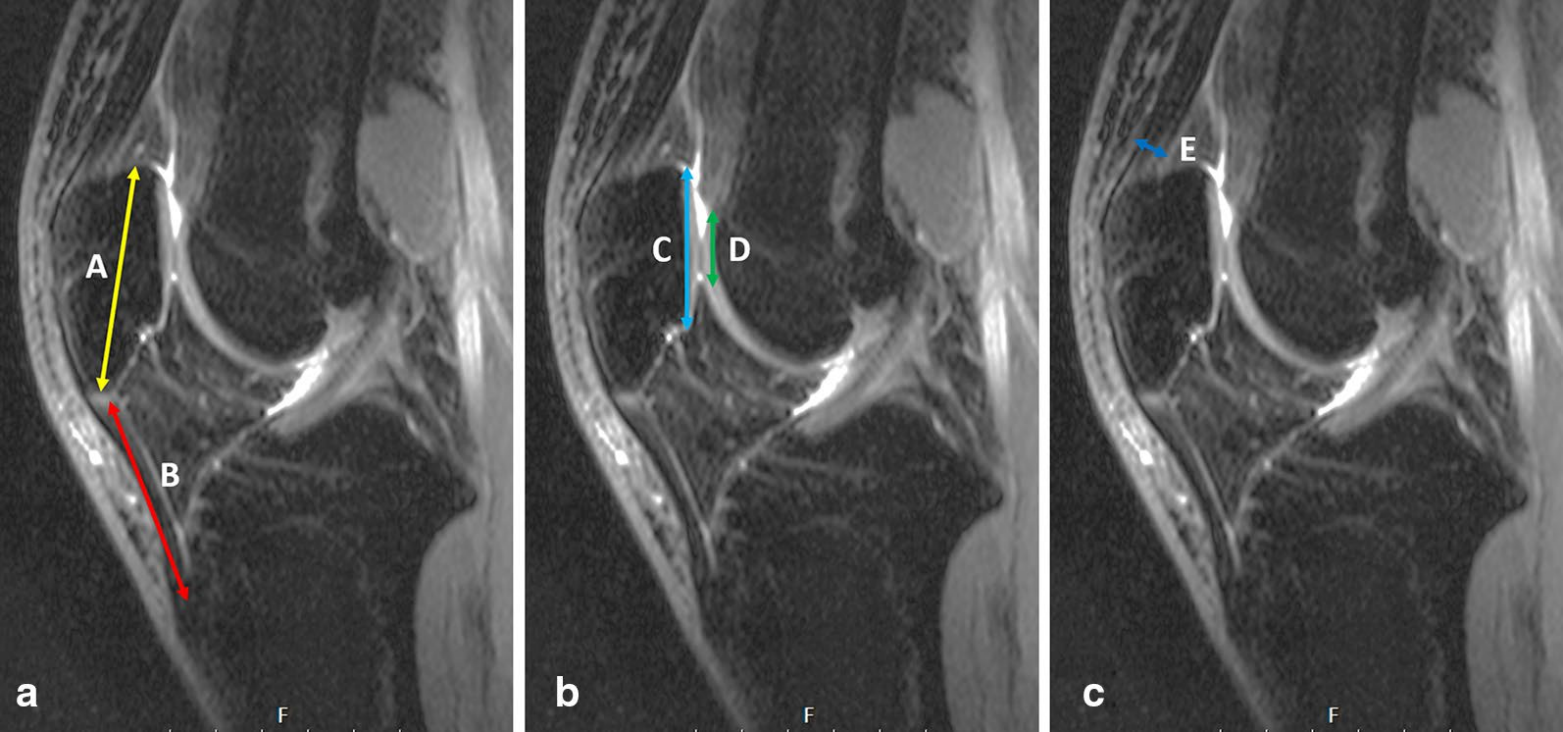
a The ratio of patellar tendon length (Line B) to longest diagonal length of patella (line A) was calculated as the Insall–Salvati ratio. b The ratio of length of articular surface of patella (line C) to length of opposing femoral articular surface (line D) was calculated as patellotrochlear index. c The distance between the most anterior and most posterior border of the quadriceps tendon just over the patellar attachment was measured and recorded as quadriceps tendon thickness. All measurements were performed on sagittal fat-saturated, proton-density-weighted MRI
